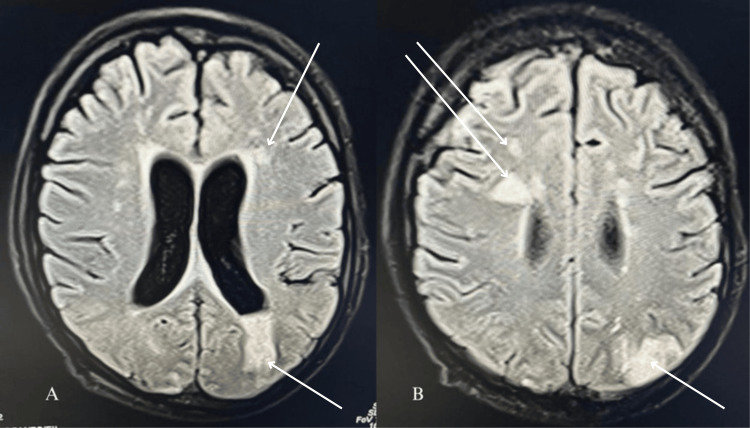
MRI flair sequence showing multiple septic emboli in the brain.
